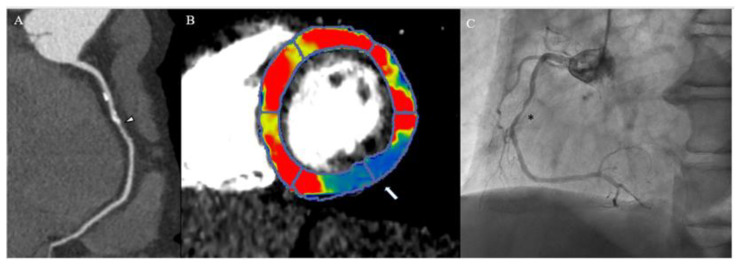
A 65-year-old male patient with a history of chest pain underwent a coronary computed tomography angiography, showing a stenosis of mid-right coronary artery (arrowhead, panel (A)). Computed tomography myocardial perfusion showed a perfusion defect of inferolateral and inferior walls marked as a blue region in comparison to normal perfused myocardium in red and yellow (arrow, panel (B)). Invasive coronary angiography confirmed a severe stenosis of right coronary artery (asterisk, panel (C)).
